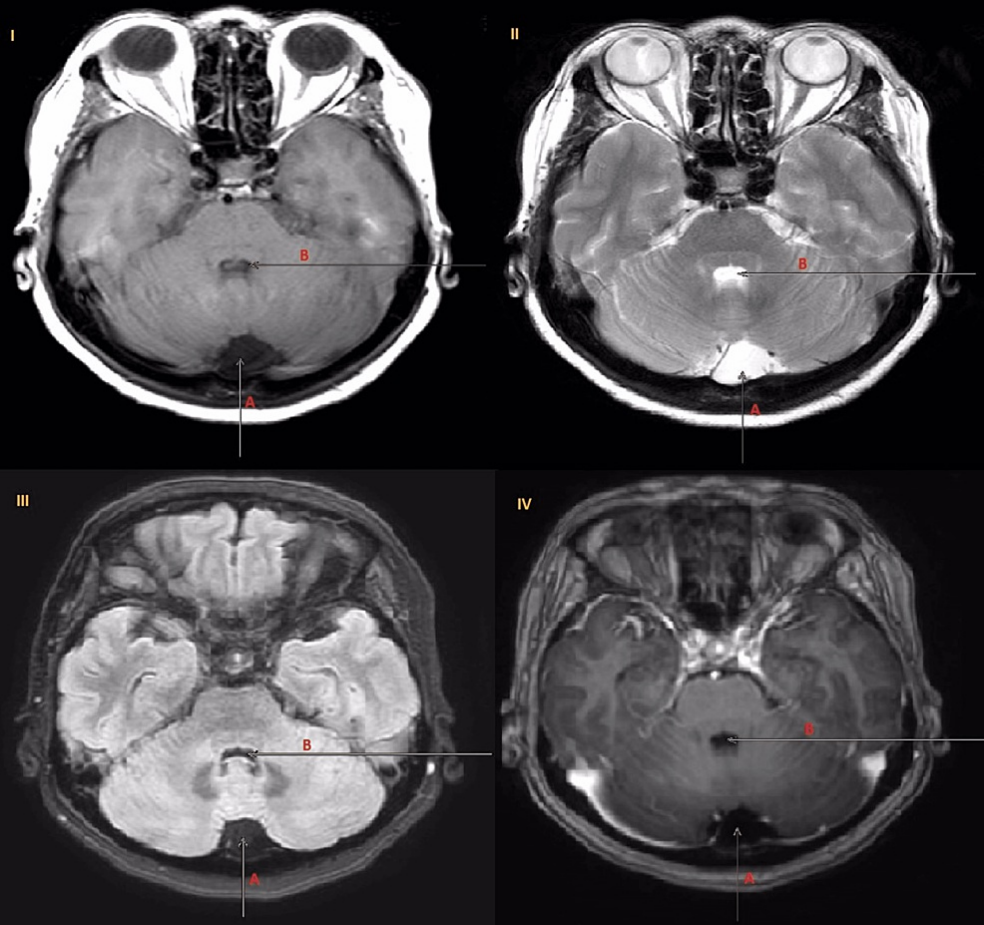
A cystic lesion in the posterior fossa (arrow A) with similar signal intensity as the CSF in the fourth ventricle (arrow B) shown in MRI in different views. I: T1-weighted image, II: T2-weighted image, III: Flair sequence image, IV: Post-gadolinium image
